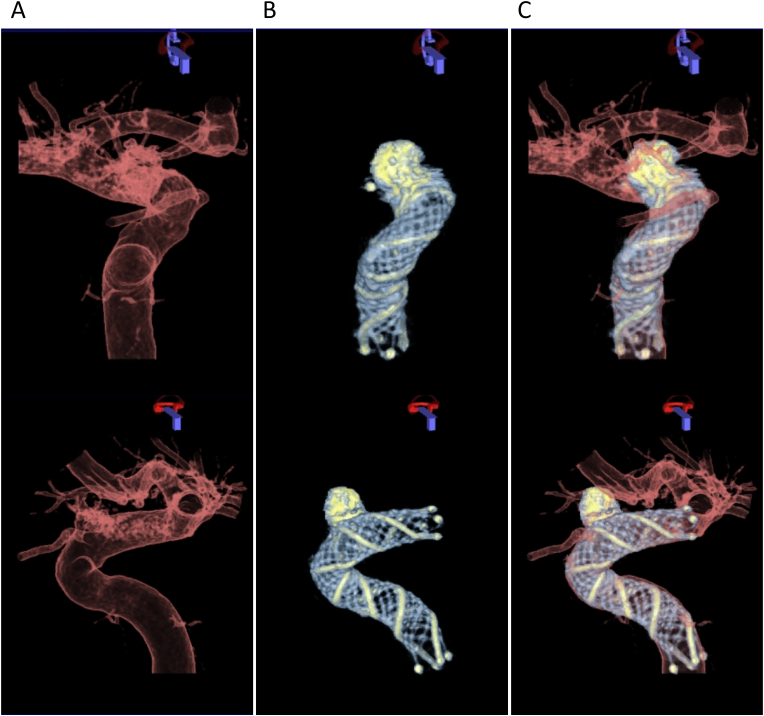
Step-by-step reconstruction of dual-volume three-dimensional (3D) fusion images. 3D vessel images acquired from 3D digital subtraction angiography. (A) (Upper) anterior-posterior and (lower) lateral view. 3D images of low-profile visualized intraluminal support (LVIS) stent generated from high-resolution cone-beam computed tomography. (B) (Upper) anterior-posterior and (lower) lateral view. Dual-volume 3D fusion images created from the 3D vessel images and the 3D stent images. (C) (Upper) anterior-posterior and (lower) lateral view.
